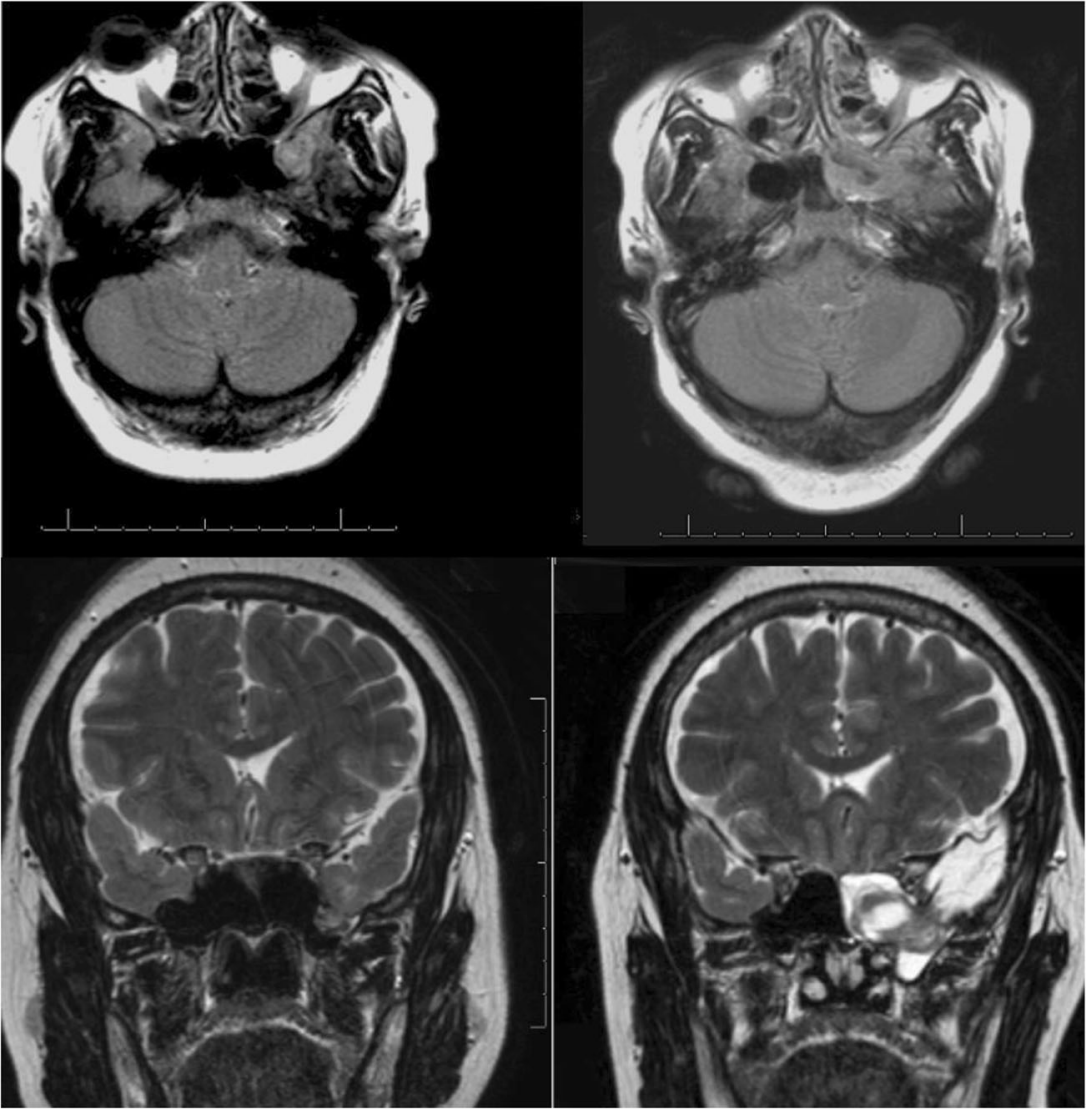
T2-weighted MRI of the brain. The MRI on the* left* is from 2005, and the one on the* right* is from 2015. They show the interval enlargement left-sided basal transsphenoidal encephalocele with associated encephalomalacia within the temporal lobe
